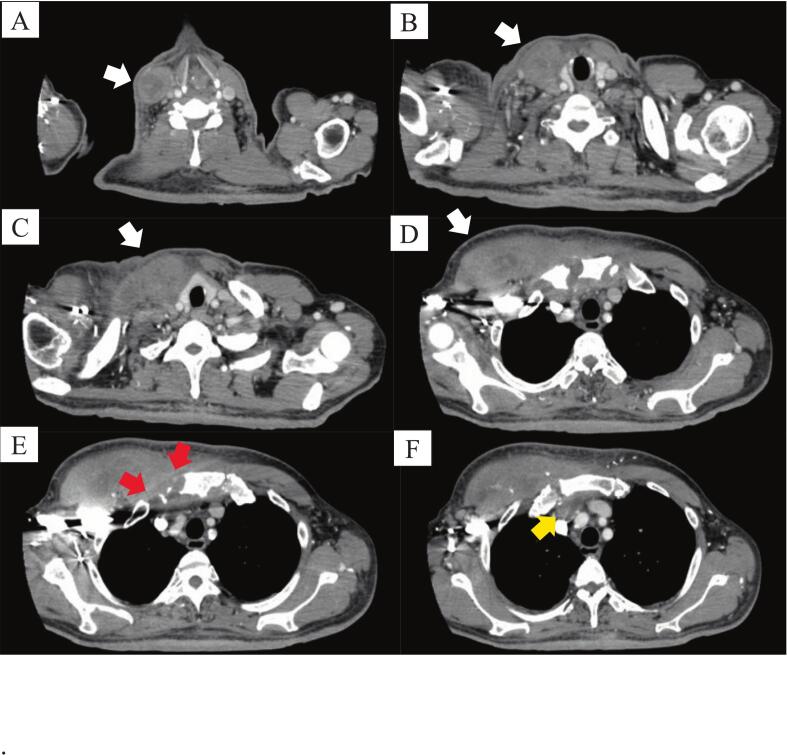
(A-F). Chest computed tomography findings. White arrows showing the cervical and anterior chest wall abscesses. Red arrows showing the destruction of the right sternoclavicular joint. A yellow arrow showing the retrosternal abscess. (For interpretation of the references to colour in this figure legend, the reader is referred to the web version of this article.)
